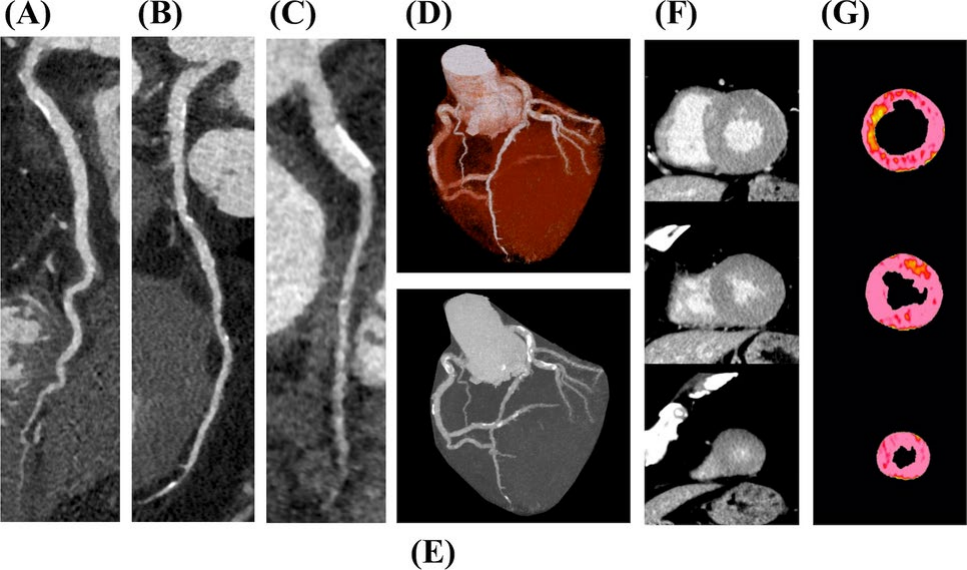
Cardiac CT images of a 70-year-old male who had not experienced MACE during a follow-up period of 5.1 years. A Curved multiplanar reconstruction of the right coronary artery, B curved multiplanar reconstruction of the left anterior descending coronary artery, C curved multiplanar reconstruction of the left circumflex coronary artery, of which CAD-RADS score was 2, indicating the absence of obstructive CAD with calcium score of 869, D volume rendering image of the heart and coronary arteries, E: maximum intensity projection image of the heart and coronary arteries, F delayed enhancement images from base, mid, and apex, and G MBF maps from base, mid, and apex. The global MBF of the patient was 150.0 ml/g/min; *MACE* major adverse cardiac event, *CAD-RADS* Coronary Artery Disease-Reporting and Data System, *CAD* coronary artery disease, *MBF* myocardial blood flow
